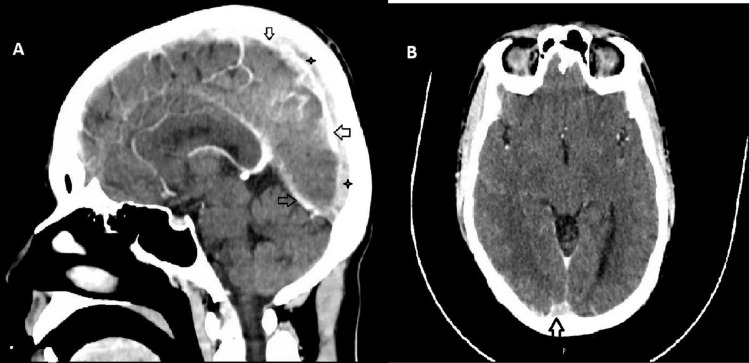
CT scan. (A) Sagittal view. (B) Axial view. Superior sagittal sinus and its tributaries show high density (arrow) with filling defect (star) on post-contrast examination suggesting dural sinus thrombosis.
